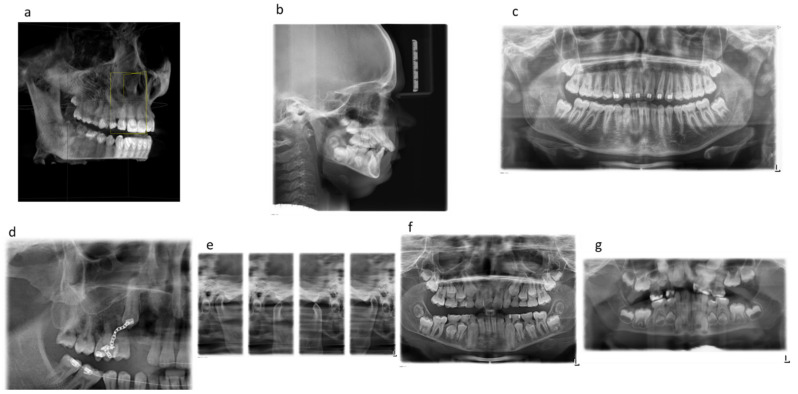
Shows examples of the extraoral dental X-ray images analyzed (Figure 1). Examples of the extraoral X-ray images analyzed: (a) CBCT, (b) lateral cephalogram, (c) OPG standard, (d) OPG without TMJ left and half OPG image, (e) OPG TMJ program, (f) OPG without TMJ right and left, (g) OPG child.
